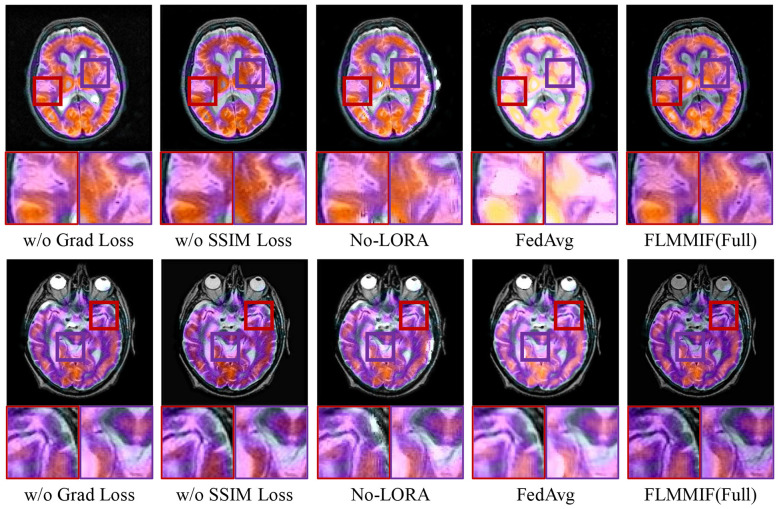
Quantitative assessment of ablation study on SPECT-MRI dataset.
